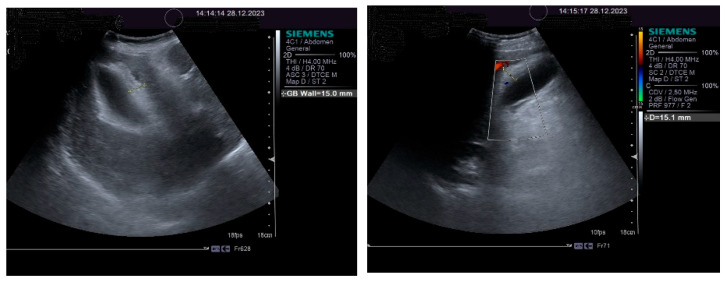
Thickening of gallbladder wall: 2D aspect (left); color Doppler aspect (right).
